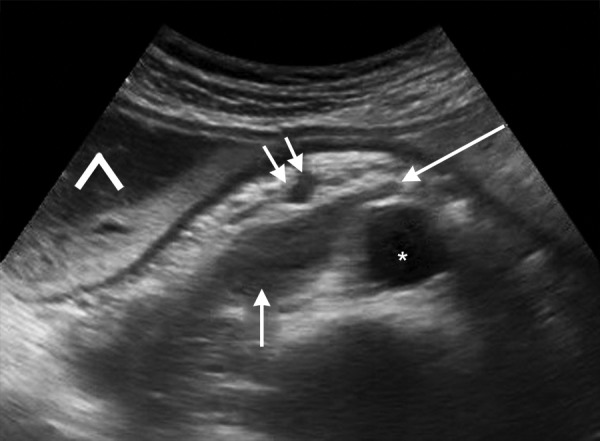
A 61-year-old male presented with symptoms of upper GI obstruction. Ultrasound of abdomen shows distension of stomach (^A^) and duodenum (short single arrow) due to compression of 3rd part of duodenum (long arrow) between SMA (double arrows) and aorta (*)
